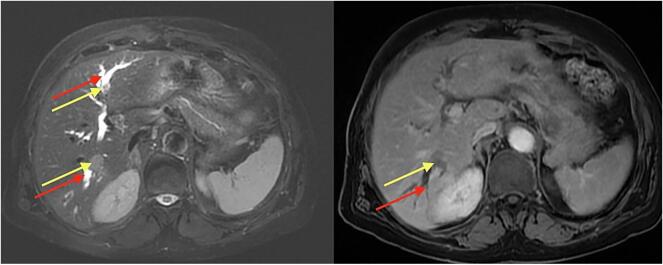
MRI of the liver with and without gadolinium injection showing a dilation of biliary tracts (red arrow) upstream two endoluminal biliary nodules in segments VII and II (yellow arrow). (For interpretation of the references to colour in this figure legend, the reader is referred to the web version of this article.)
